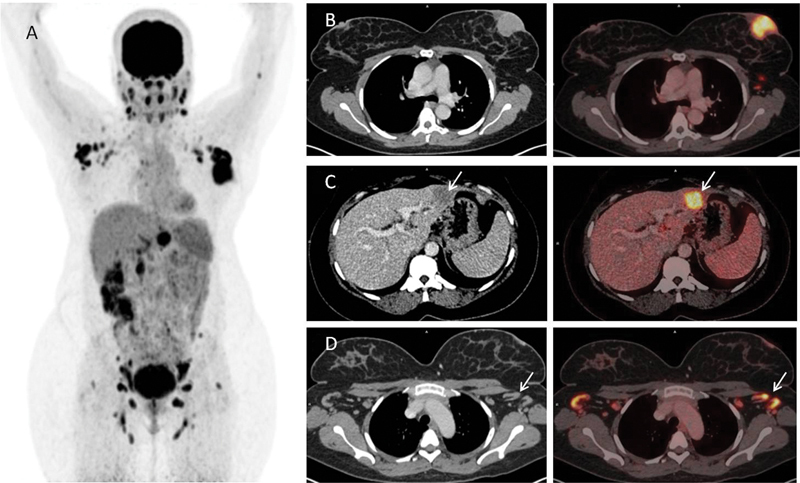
18F-FDG PET/CT : Maximum intensity projection, Axial CT and axial fused PET/CT images (From Left to Right).
